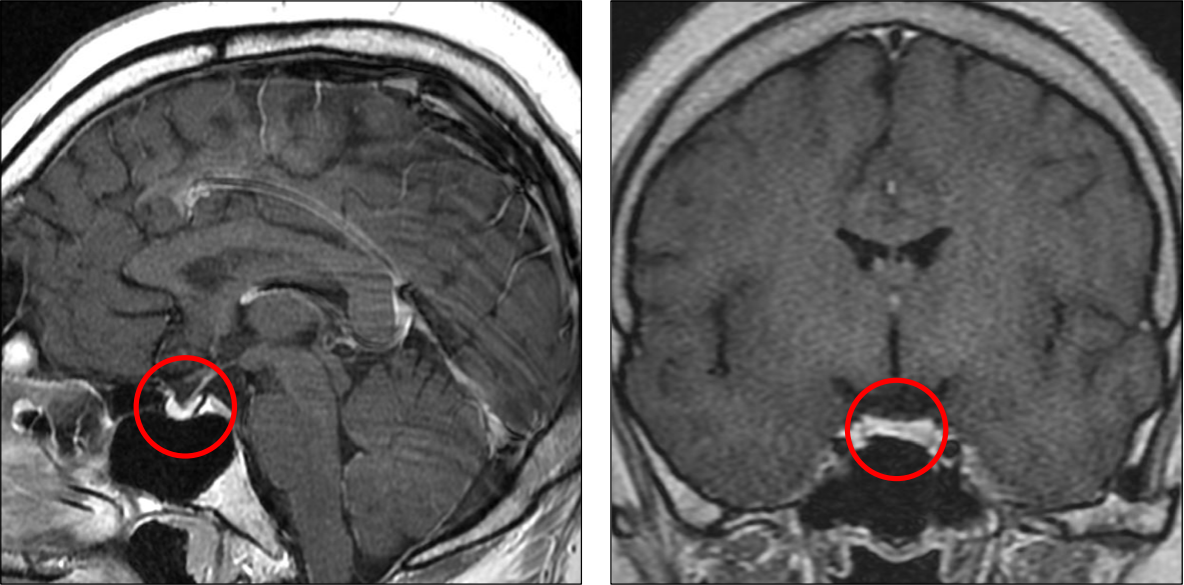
Gadolinium-enhanced brain magnetic resonance imaging showing anterior pituitary hypoplasia (circle)
